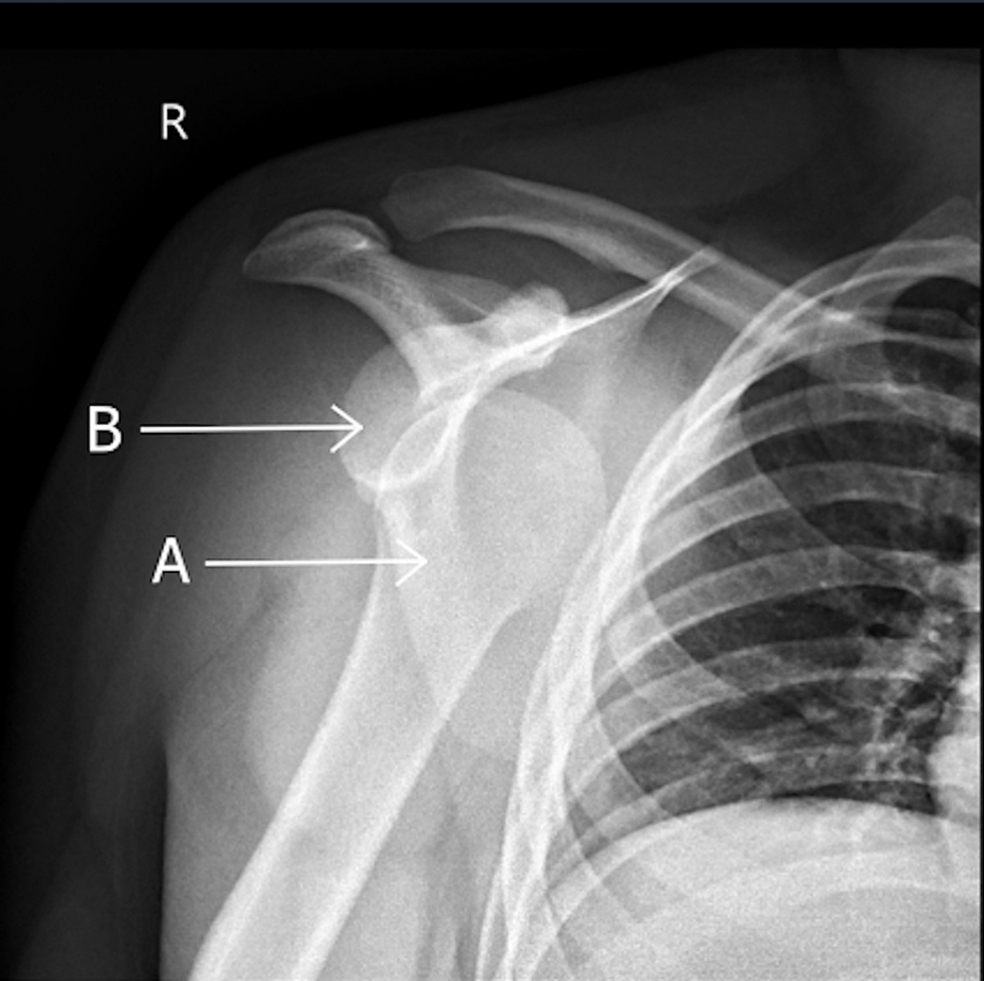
Anterior-posterior X-ray showing the anteriorly dislocated right glenohumeral joint. The head of the humerus (A) is dislocated anterior to the glenoid (B).
